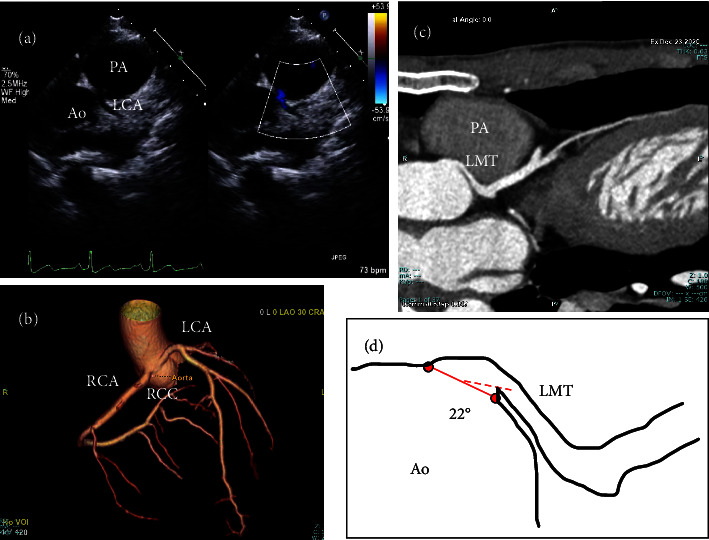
(a) Transthoracic echocardiography (parasternal short axis) at initial examination. The origin of pain in the left coronary artery is not detected in its normal position. (b) Coronary computed tomography angiography (CCTA, three-dimensional reconstruction) depicting the left coronary artery originating from the right sinus of Valsalva. (c) CCTA shows that part of the left main coronary artery trunk runs between the aorta and pulmonary artery. (d) Measurements of the take-off angle from the ascending aorta in the left coronary artery. The solid line indicates the so-called ostium line, and the dashed line indicates the line drawn from the midpoint of the ostium line to a point along the center of the left main trunk. The angle between the two lines at the midpoint is measured as the take-off angle and is 22 degrees. Ao: aorta; PA: pulmonary artery; LCA: left coronary artery; RCA: right coronary artery; RCC: right coronary cusp; Left main coronary trunk.
